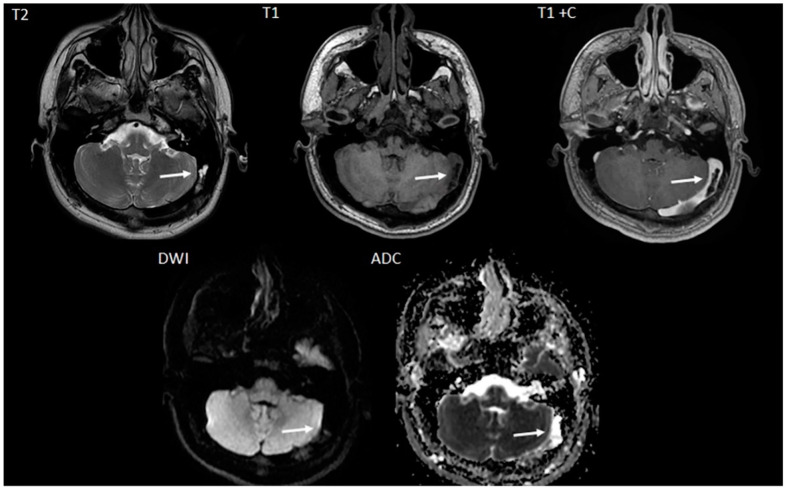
Axial T2-weighted (T2), T1-weighted (T1), T1-weighted with contrast (T1+C), diffusion weighted image (DWI) MRI images, and apparent diffusion coefficient (ADC) map reveal a CSF isointense, T2-hyperintense giant arachnoid granulation matching the CT finding (arrows). On the contrast enhanced sequence, contrast enhancement is noted outlining the non-enhancing Pachionic granulation. On diffusion weighted imaging (DWI) and the matching apparent diffusion coefficient (ADC) map the Pacchionian granulation is characterized by facilitated diffusion (DWI-hypointense, ADC bright) similar to CSF.
